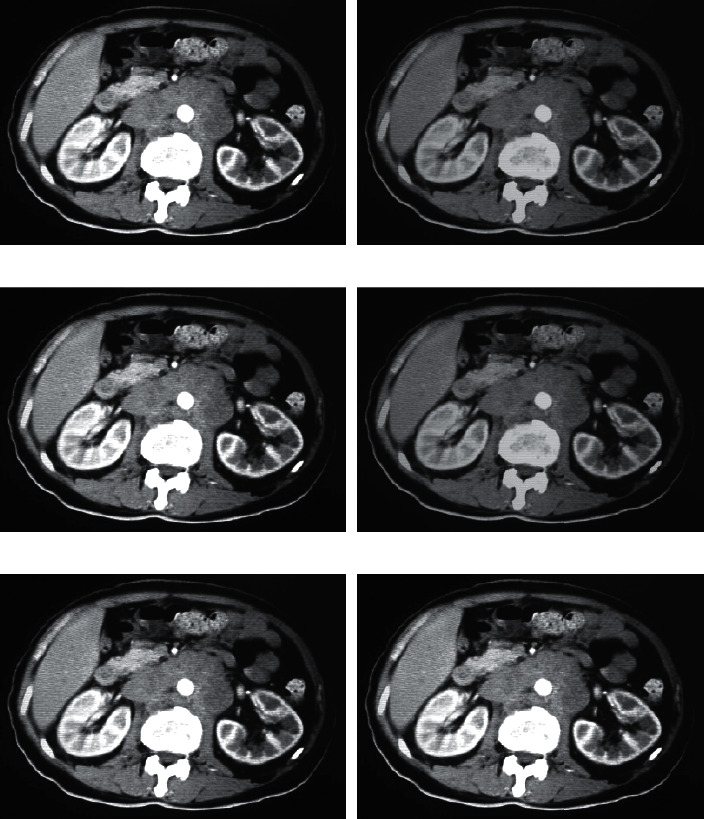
FBP and ASIR reconstruction of the CT scan of the abdomen of ICP patients. A, B, and C represent the plain scan phase, arterial phase, and portal phase, respectively, and A1, B1, and C1 represent FBP, while A2, B2, and C2 represent ASIR.
